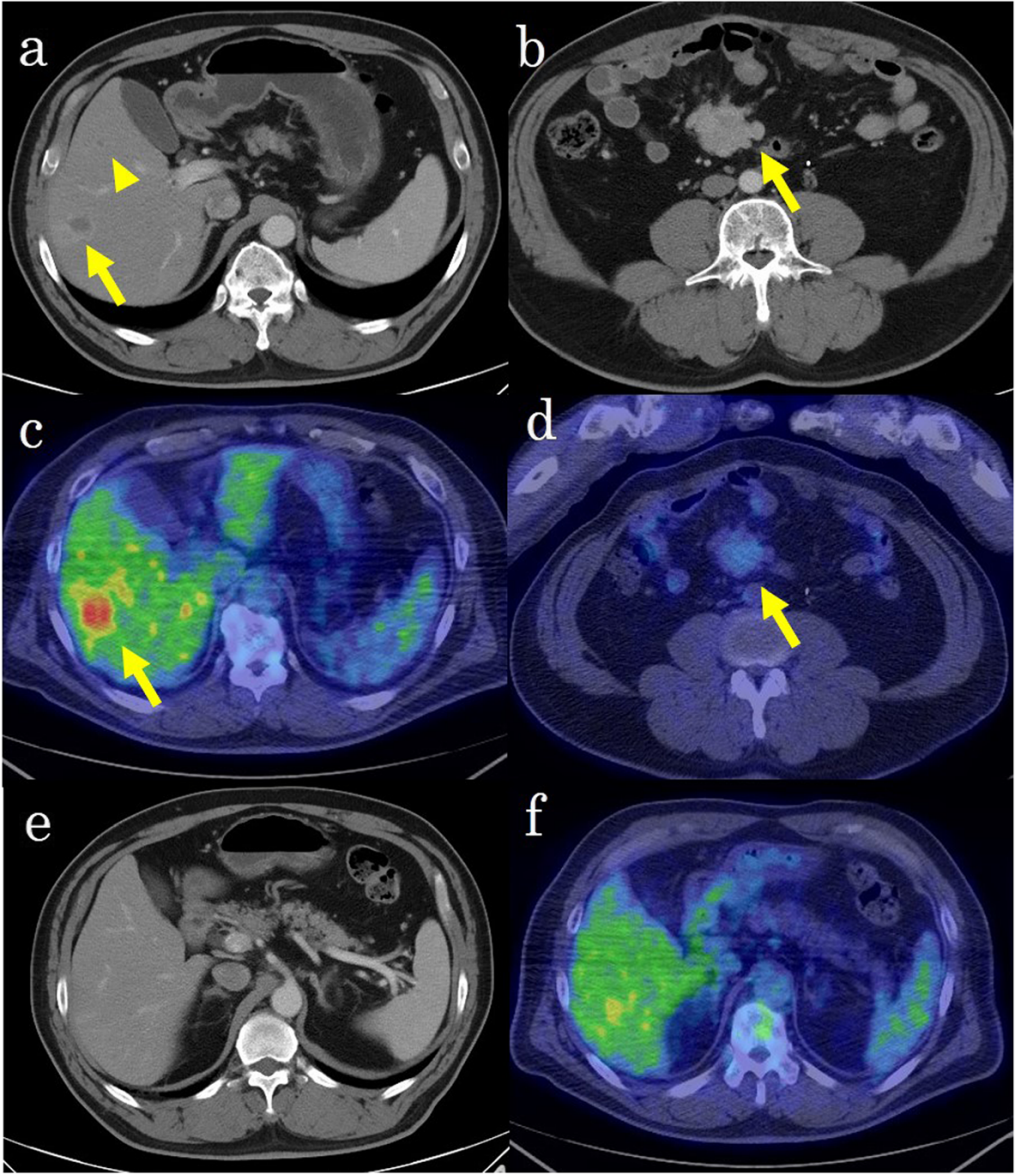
Contrast-enhanced computed tomography (CT) and [18F]-fluorodeoxyglucose positron emission tomography (FDG-PET) images 1 year after abdominoperineal resection. a Contrast-enhanced CT showed two tumors in segment 5 (arrow and arrowhead) that were enhanced in the arterial phase. b Contrast-enhanced CT showed a 47-mm tumor in the ileal mesentery (arrow) that was enhanced in the arterial phase. c On FDG-PET, accumulation of [18F]-FDG was found in segment 5 (arrow) tumor. d On FDG-PET, only a little accumulation of [18F]-FDG was found in the ileal mesenteric tumor (arrow). e Contrast-enhanced CT showed no tumor in segment 6 that was enhanced in the arterial phase. f On FDG-PET, no accumulation of [18F]-FDG was found in segment 6
